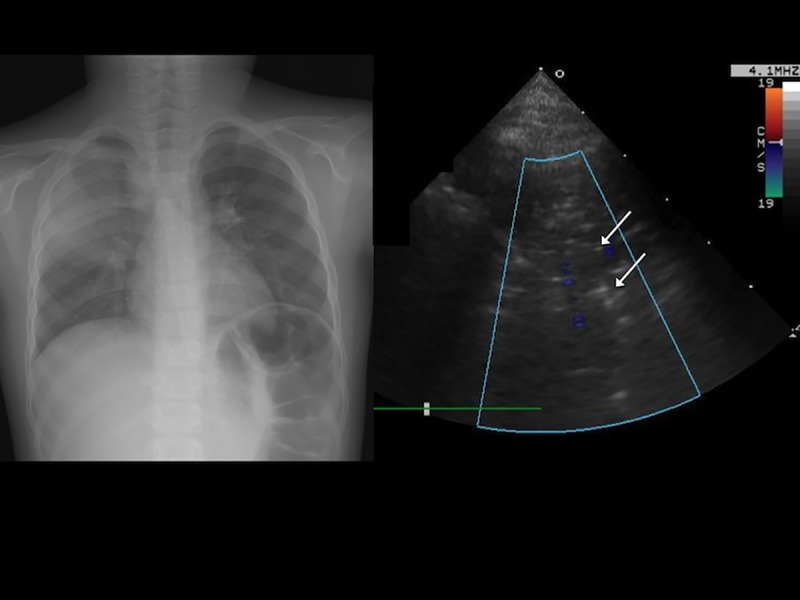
A 5-year-old boy with community-acquired pneumonia classified as severe pneumonia in our study. (A) Chest radiography revealed right upper lobe consolidation. (B) Transthoracic ultrasonography revealed multiple anechoic tubular structures without a color Doppler signal in the right anterior upper lung, indicating the presence of a fluid bronchogram (arrows).
